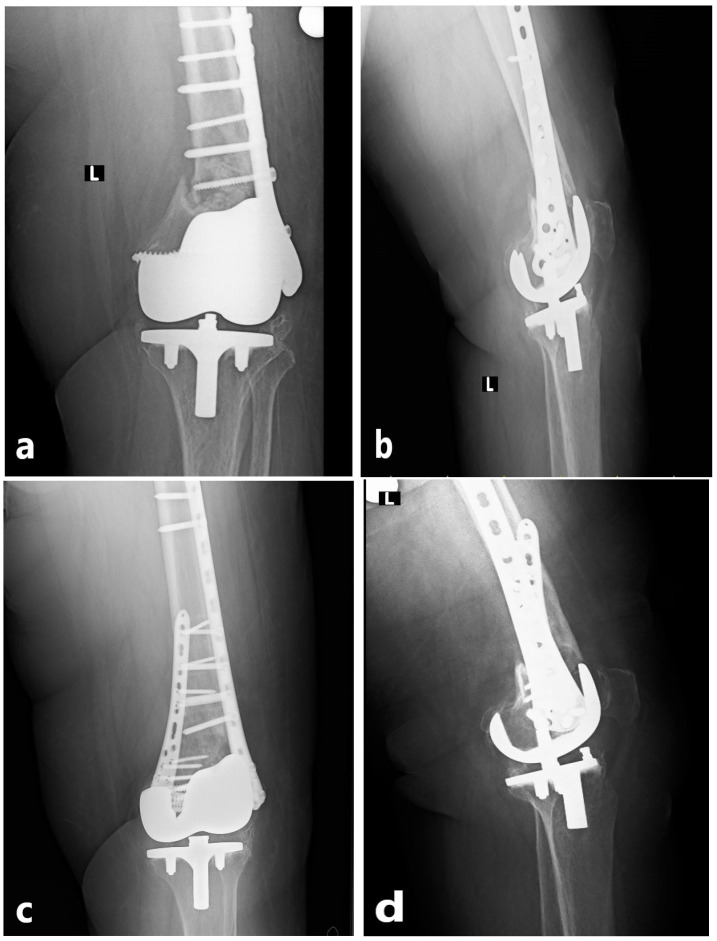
Radiographic images of a 76-year-old female patient. (a,b) Anteroposterior and lateral views demonstrating implant failure after the initial fixation of a periprosthetic distal femur fracture. (c,d) Anteroposterior and lateral radiographs at postoperative 3 months following revision with double-plate fixation. The radiographic marker “L” indicates the left extremity.
